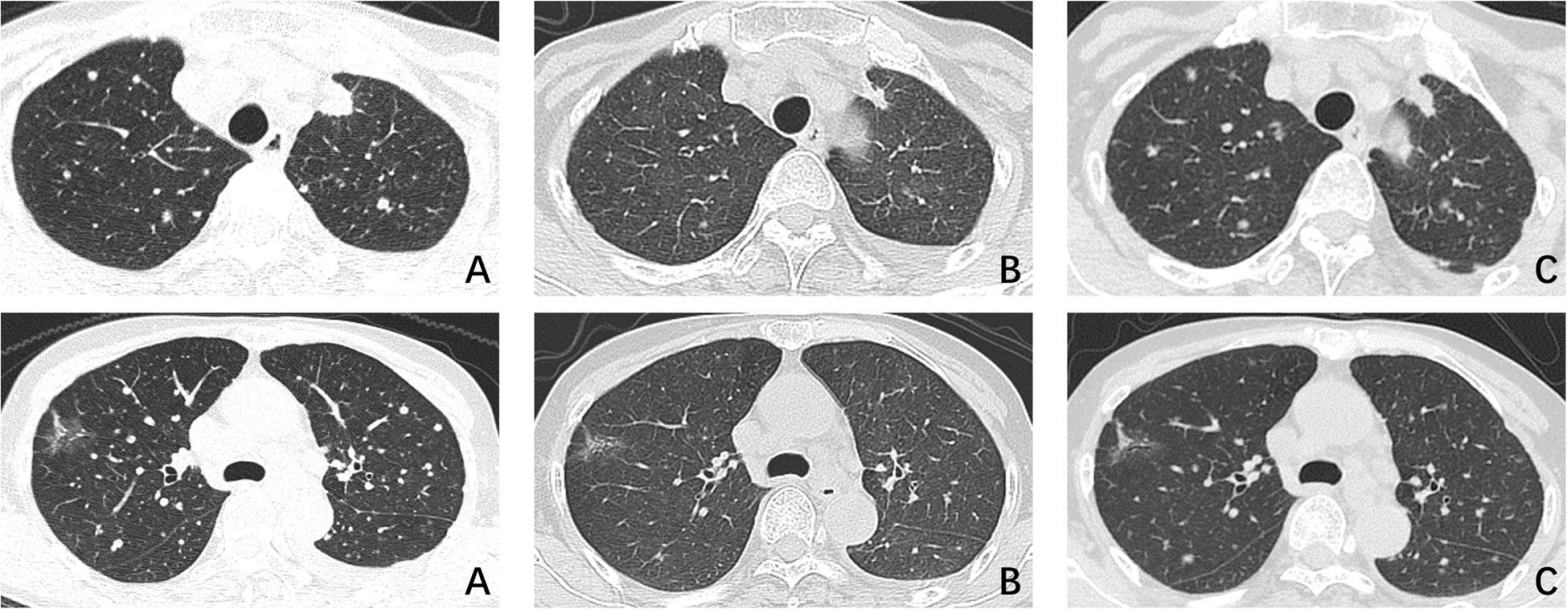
CT scans of chest during different period of treatment a Chest CT scan showed a 1 cm × 1 cm irregular nodule in the upper lobe of left lung, with diffuse bilateral pulmonary metastases. b Bilateral nodules evidently shrunk after treatment with afitinib for 3 months. c Lesions of lung progressed after ten months of targeted therapy
